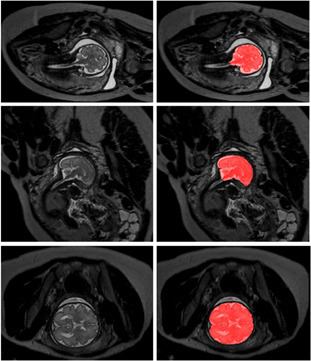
An example demonstrating the use of a U-net to automatically segment the fetal brain from a fetal MRI study. *T*
